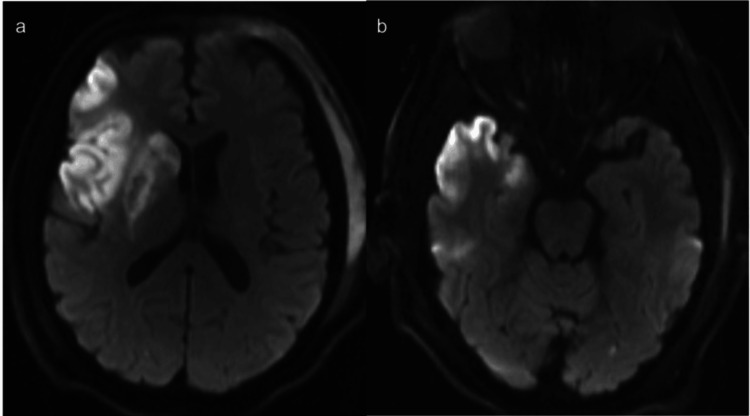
Diffusion-weighted MRI performed 24 hours after thrombectomy showing infarcts in the right basal ganglia and frontal lobe (a) and right temporal lobe (b)
